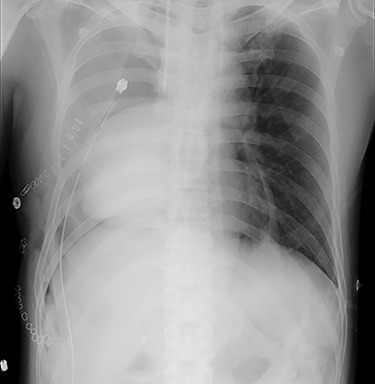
Chest radiography showing cardiac herniation into the right-sided thoracic cavity.
